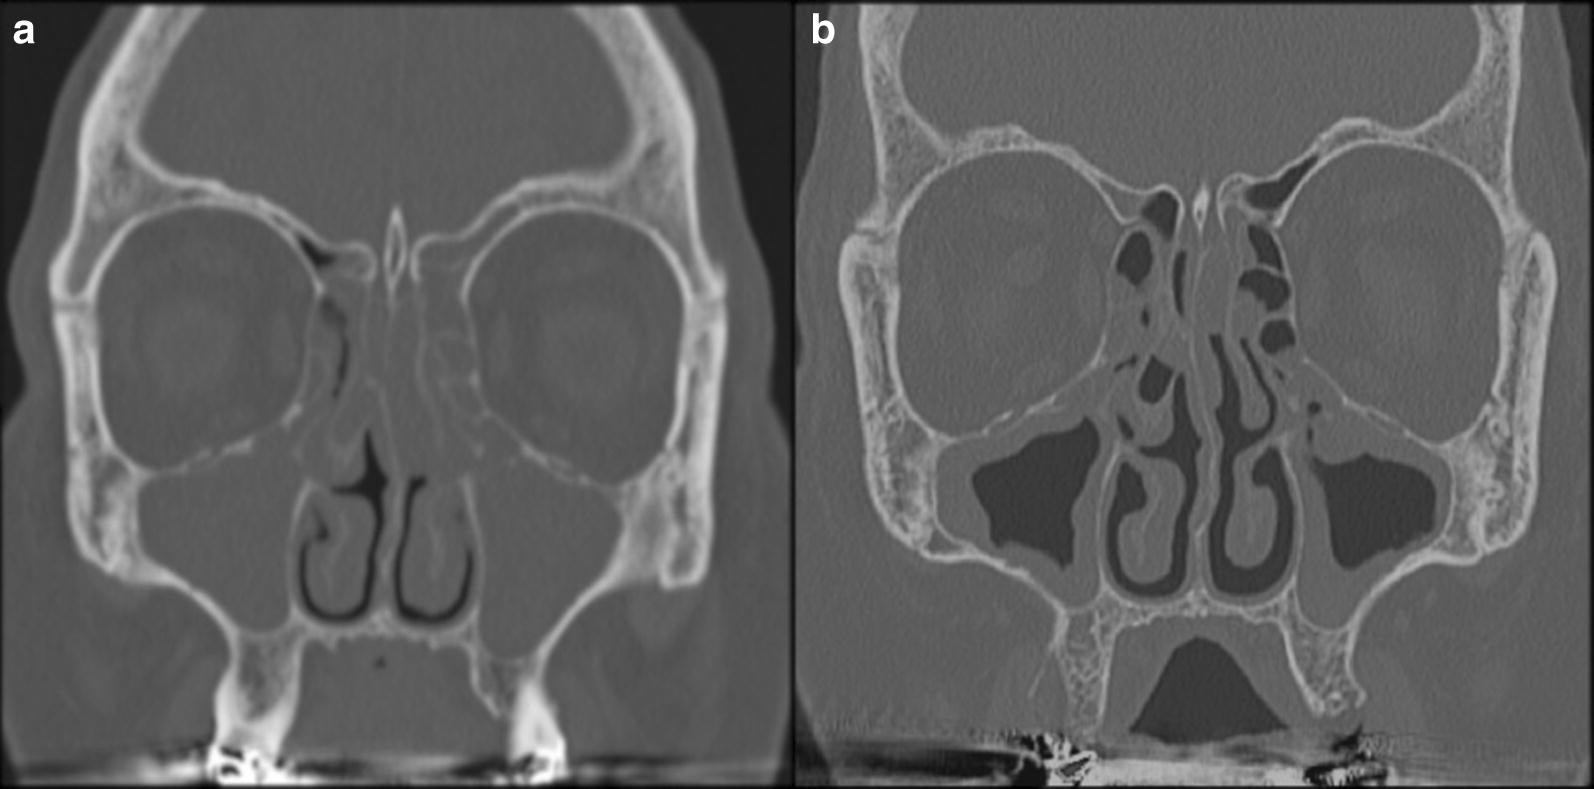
Coronal CT comparison before and after therapy: paranasal sinuses in coronal projection at T0: engagement of both maxillary sinuses, nasal cavities and ethmoid (a), and at T1: visible improvement of both maxillary sinuses, with almost complete patency of the nasal cavities and the ethmoid (b)
